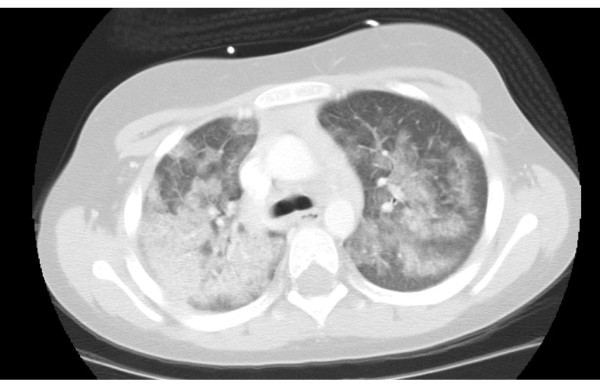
CT scan at the carina level of the lung showing hemorrhage during the second admission.
